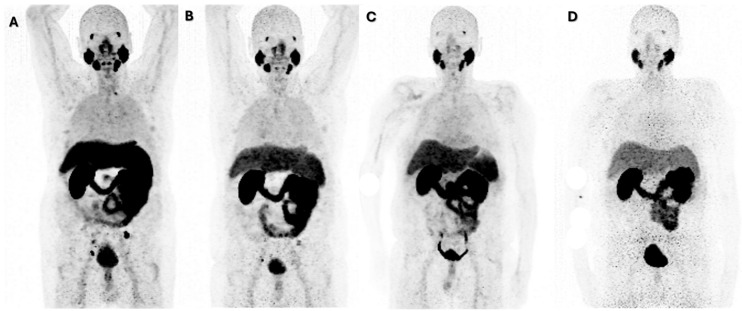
A 68-year-old male with Gleason score 7 prostate adenocarcinoma, BRCA germline negative, somatic negative. He had previous local treatment with brachytherapy and external beam radiation. The previous systemic therapy included enzalutamide and leuprorelin. A baseline [^68^Ga]Ga-PSMA PET/CT was performed in 2018, with PSA 0.70 ng/mL. The scan showed PSMA-avid bone and LNM and PSMA uptake in prostate bed (A), MIP. The patient was then treated with four cycles of [^177^Lu]Lu-PSMA PRLT. At three months post [^177^Lu]Lu-PSMA PRLT, a [^68^Ga]Ga-PSMA PET/CT was performed. The scan showed a good response in a majority of the previously seen PSMA-avid lesions in bone and soft tissue (B), MIP. A further two cycles of [^177^Lu]Lu-PSMA PRLT were administered for residual disease and a [^68^Ga]Ga-PSMA PET/CT was performed six months post these additional cycles of [^177^Lu]Lu-PSMA PRLT. PSA at time of scan was 0.10 ng/mL. The scan revealed a complete response (C), MIP. A follow-up [^68^Ga]Ga-PSMA PET/CT was performed at one year post six cycles of [^177^Lu]Lu-PSMA PRLT. The scan demonstrated persistent improvement with no PSMA-avid lesions and corresponding undetectable PSA (<0.02 ng/mL) (D), MIP. Hormonal therapy was subsequently discontinued. The patient remained in remission clinically, symptomatically, biochemically and on molecular imaging.
